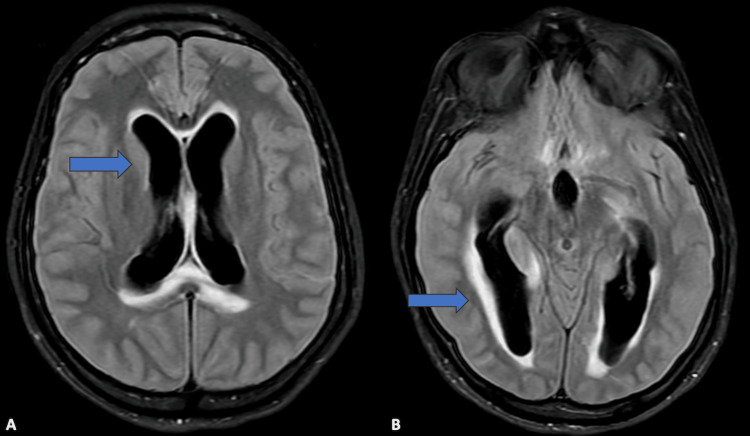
Axial fluid-attenuated inversion recovery (FLAIR) images showing triventricular dilatation (A) with transependymal CSF flow (B).
